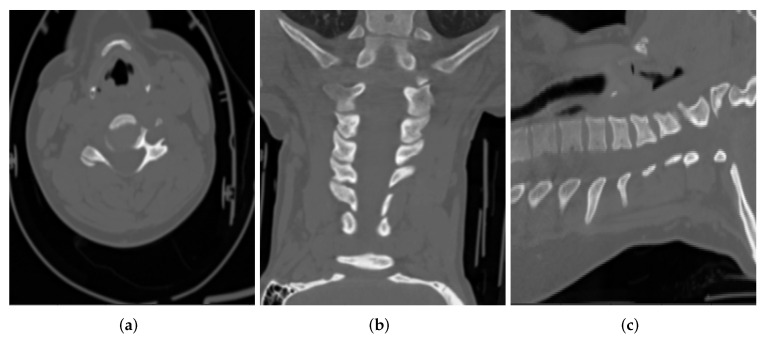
Sample CT images from (a) Axial, (b) Coronal and (c) Sagittal views for a patient.
